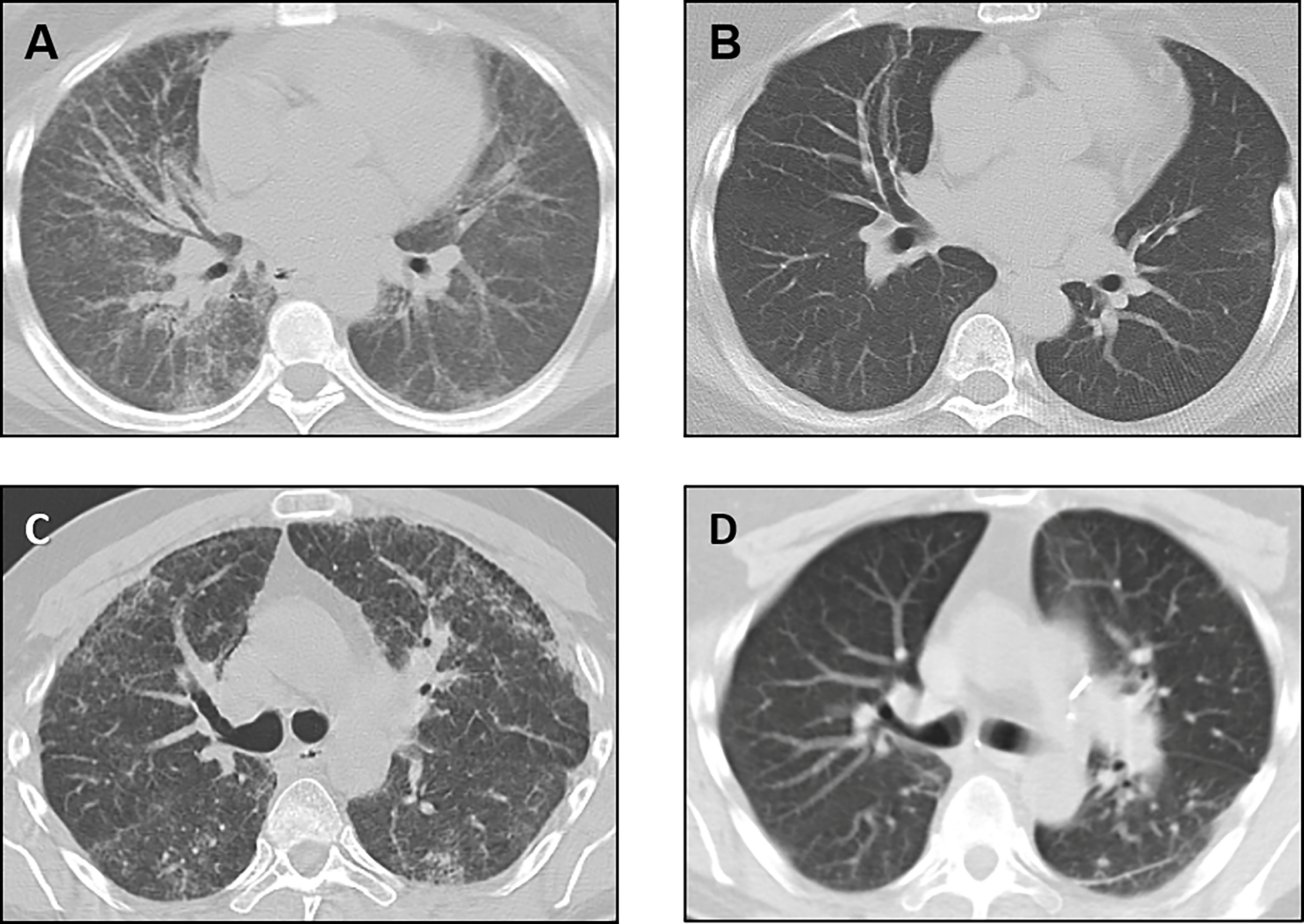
Chest computed tomography scans in Hermansky-Pudlak syndrome pulmonary fibrosis patients before and after lung transplantation. Representative computed tomography scan images of the chest from one patient with Hermansky-Pudlak syndrome pulmonary fibrosis showing diffuse bilateral interstitial infiltrates at the time of referral for lung transplantation (A) and 6 years after bilateral lung transplant surgery (B). Representative computed tomography scan images of the chest from another patient with Hermansky-Pudlak syndrome pulmonary fibrosis showing bilateral pulmonary fibrosis before lung transplantation (C) and 1.5 years after bilateral lung transplant surgery (D). Post-operative surgical changes are found, but there is no radiographic evidence of recurrence of Hermansky-Pudlak syndrome pulmonary fibrosis in the lung allografts.
